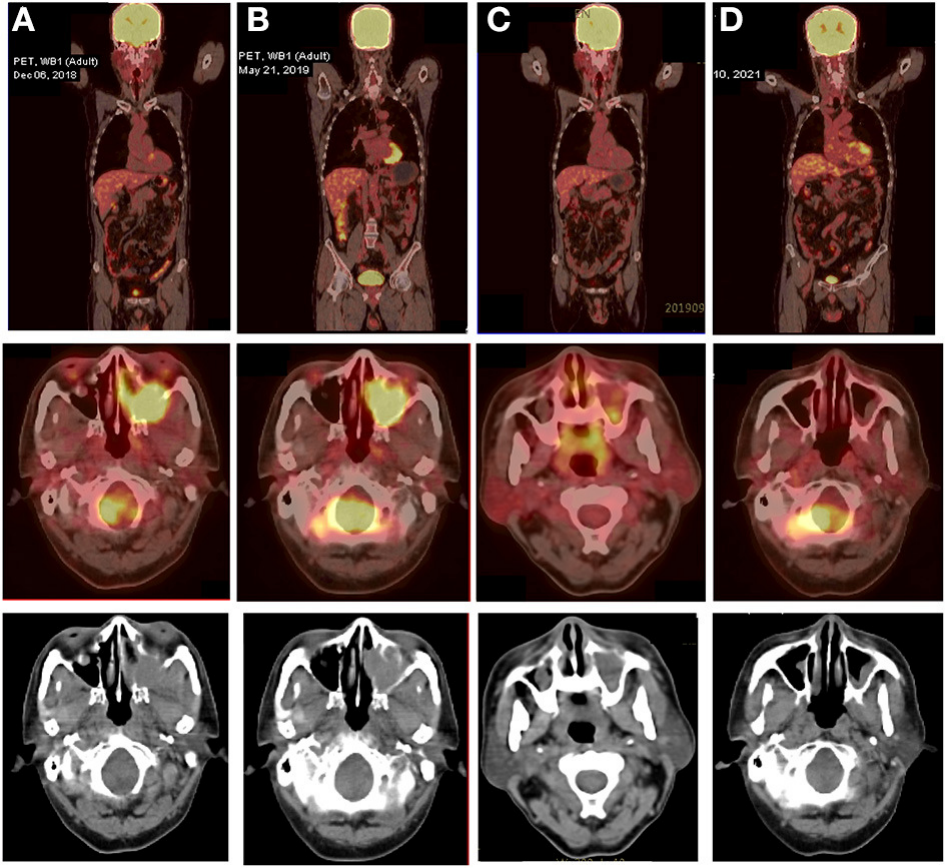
^18^F-FDG PET/CT images of the PBL patient at various stages of treatment. (A) Transverse PET/CT scan showed a markedly FDG-avid mass at the left maxillary sinus that invaded the left nasal cavity, left orbital apex, and left alar mandibular space at diagnosis (SUV_max_, 7.8; SUV_mean_, 6.3). (B) After five cycles of V-CHOP, re-evaluating PET/CT showed diminished range of lesions to 3.8 cm × 2.9 cm × 3.8 cm and reduced ^18^F-FDG uptake in the left maxillary sinus (SUV_max_, 7.0; SUV_mean_, 4.5) and other locations (SUV_max_, 4.0; SUV_mean_, 3.6). (C) After local radiotherapy and two cycles of RV-CDOP, PET/CT showed a complete metabolic response (cMR) with a diminished range of lesions to 1.0 cm × 1.3 cm and significantly reduced 18F-FDG uptake in the left maxillary sinus (SUV_max_, 3.5; SUV_mean_, 2.5) and a lack of metabolic activity in other regions. (D) Follow-up PET-CT 16 months post ASCT showed continued cMR.
